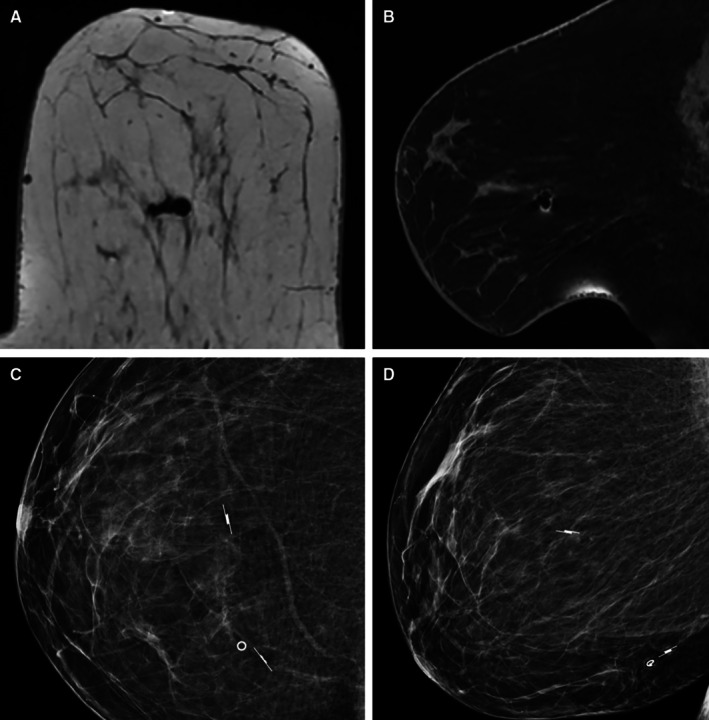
Appearance of SCOUT localiser on MRI and corresponding mammogram, inserted for non‐mass enhancement. (A) Axial 3D T1 non‐fat saturated sequence, (B) Sagittal T1 fat‐saturated non‐contrast sequence, (C) CC projection, (D) MLO projection. A second SCOUT localiser was inserted into the clipped index malignancy under ultrasound, demonstrating bracketing capability.
